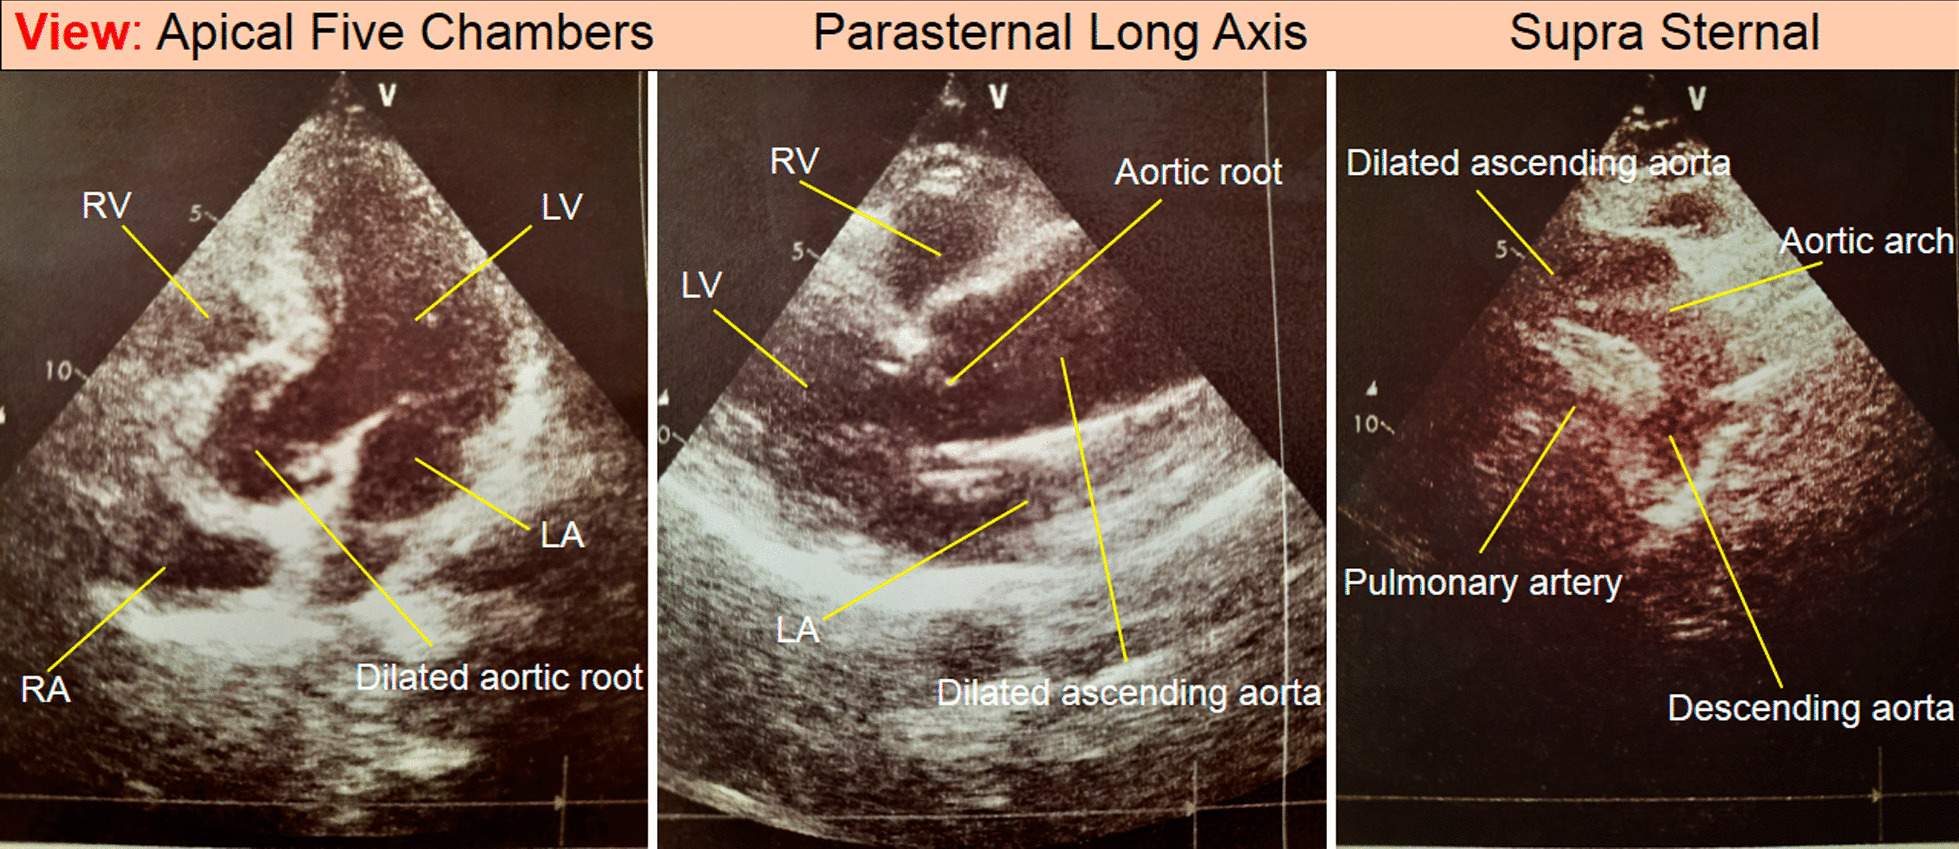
Echocardiography showed aortic aneurysms and related abnormalities
